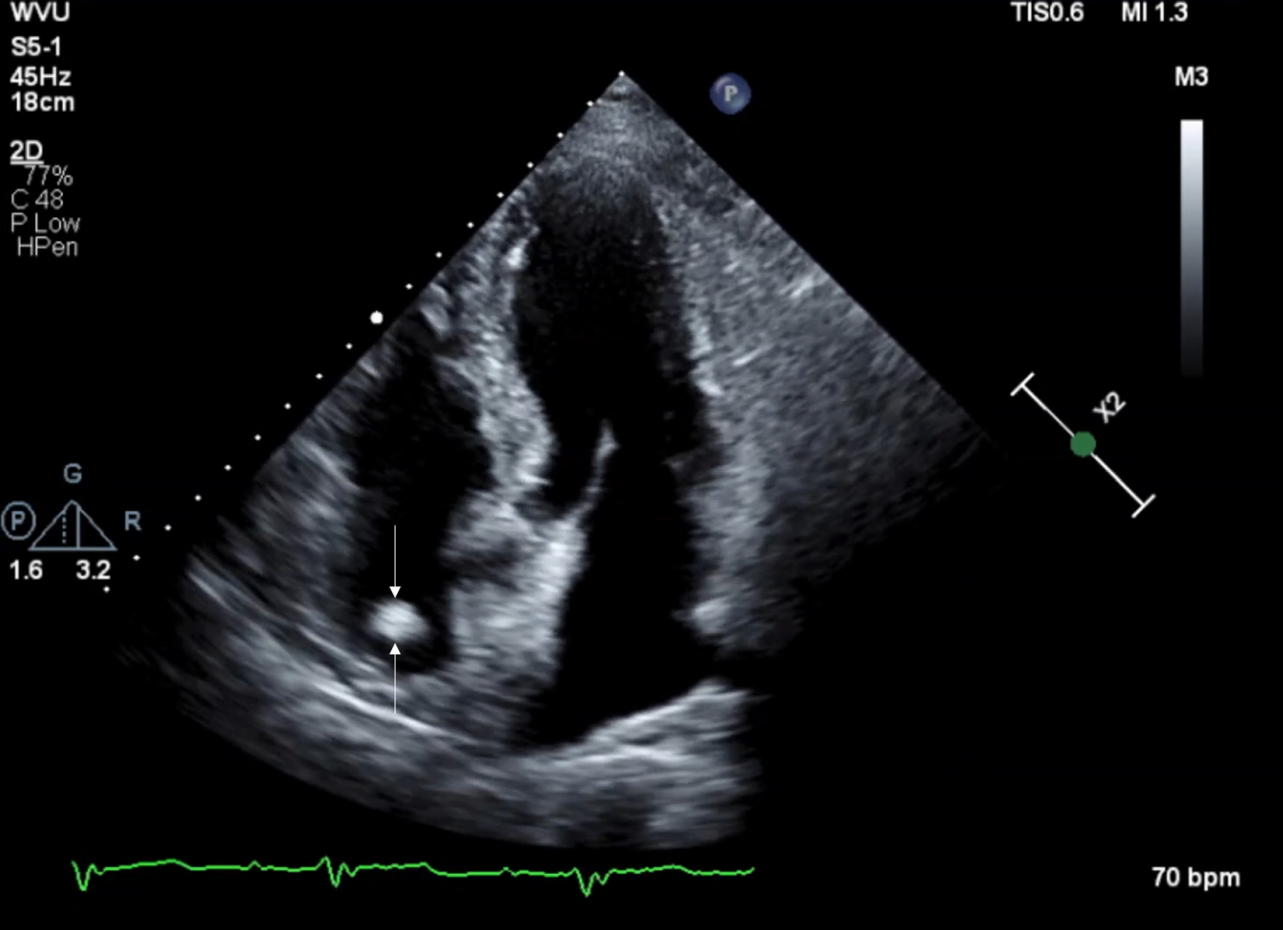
Figure 1: Transthoracic echocardiogram. Transthoracic echocardiogram was performed as a part of preoperative workup prior to surgery. Four-chamber transthoracic echocardiogram shows a prominent crista terminalis (arrow) in the right atrium, which was initially reported as indeterminate, and concerning for malignancy. Further evaluation with CT or MRI cardiac morphology was recommended
